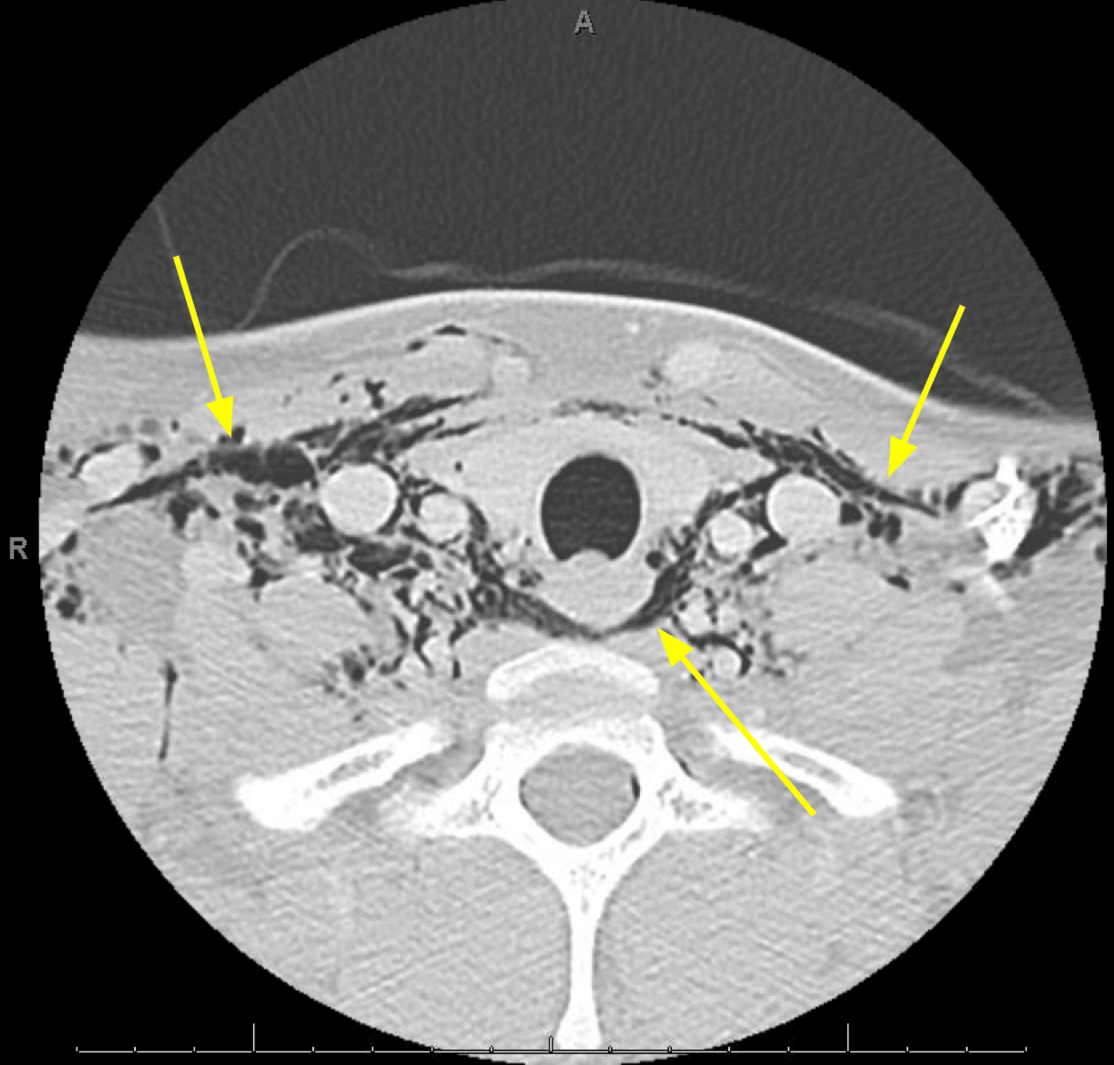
Axial computed tomography with contrast of the neck and soft tissues at the thoracic inlet showing subcutaneous emphysema within the neck
